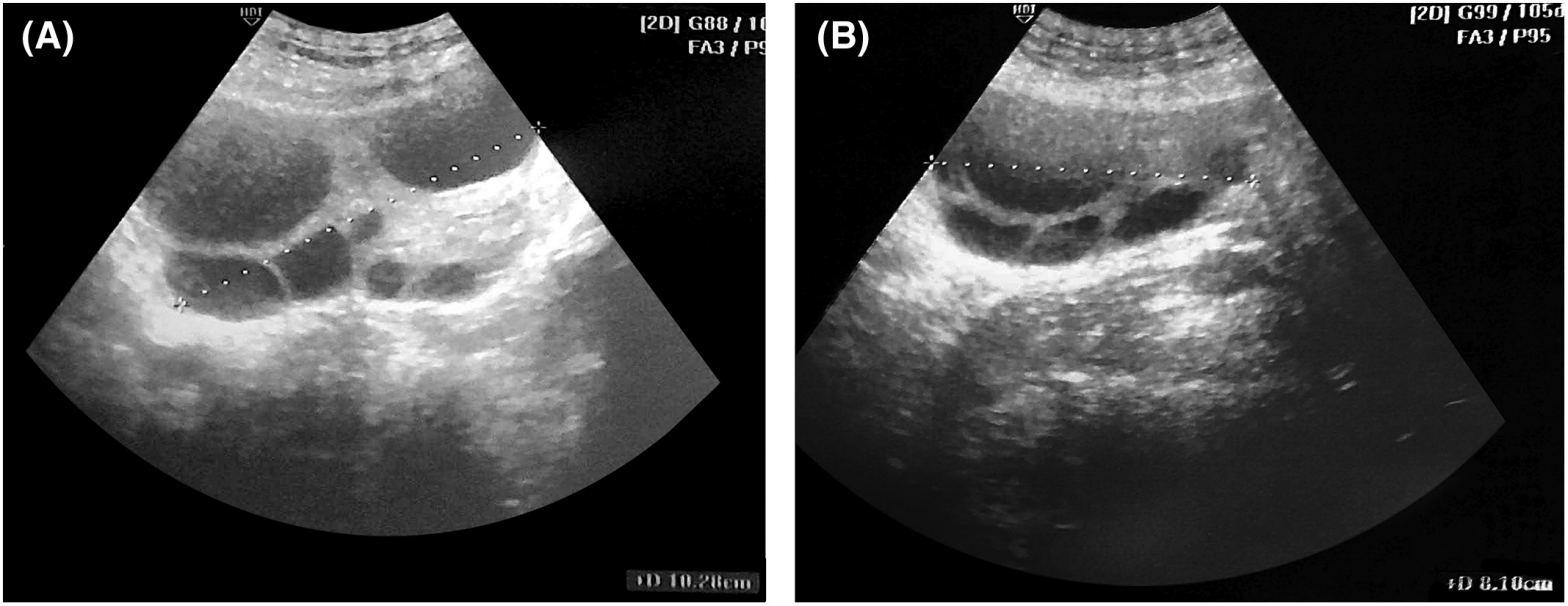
Ultrasound follow‐up eight weeks after delivery. The maximal diameter of the ovaries shrank to 10.3 cm on the right side (A) and 8.1 cm on the left side (B). The cystic complex shows an obvious regression in comparison with Figure 1B.
